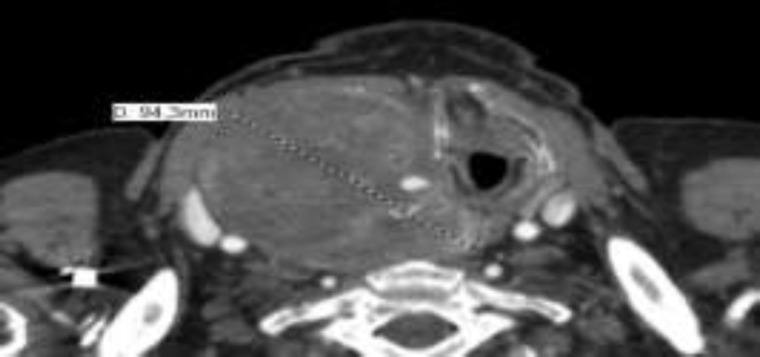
CT scan image showing a giant mass with narrowing and contralateral displacement of larynx and trachea
